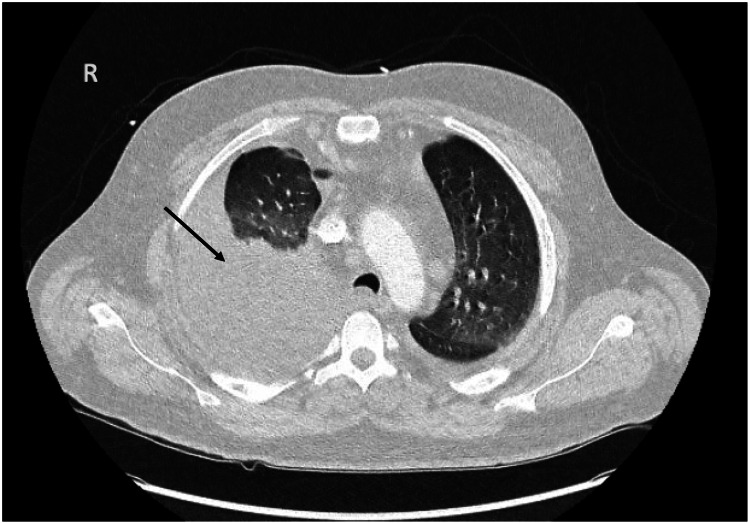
CT of the chest with a large right pleural effusion (arrow). CT: computed tomography
